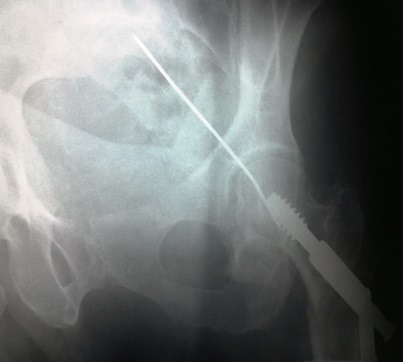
Radiographie du bassin montrant la broche en situation intra-pelvienne (observation 3)
